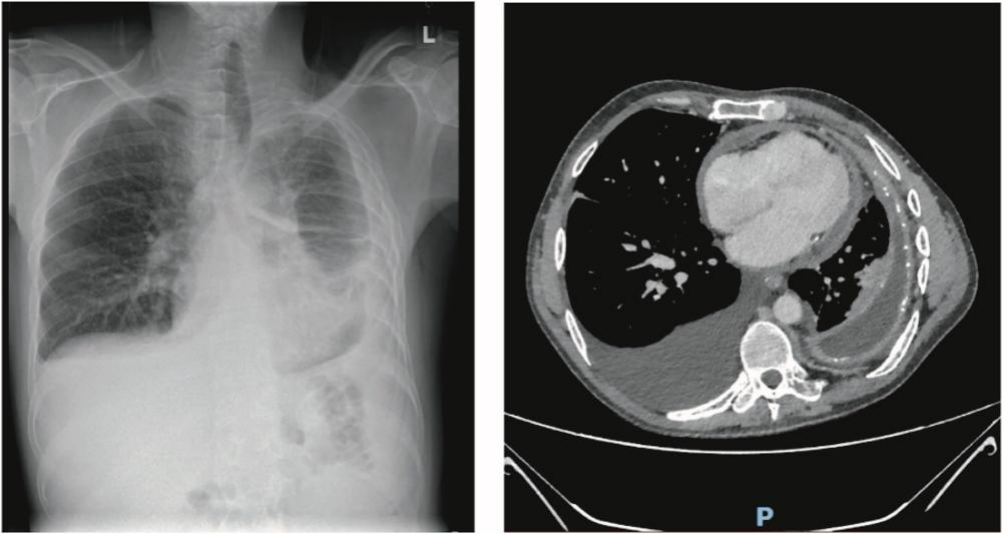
Index admission chest radiograph (*A*) demonstrating bilateral pleural effusions and left mid/lower zone opacification. (*B*) Axial slice of contrast computed tomography scan demonstrating rim enhancing pericardial effusion and bilateral pleural effusion with left-sided pleural calcification.
